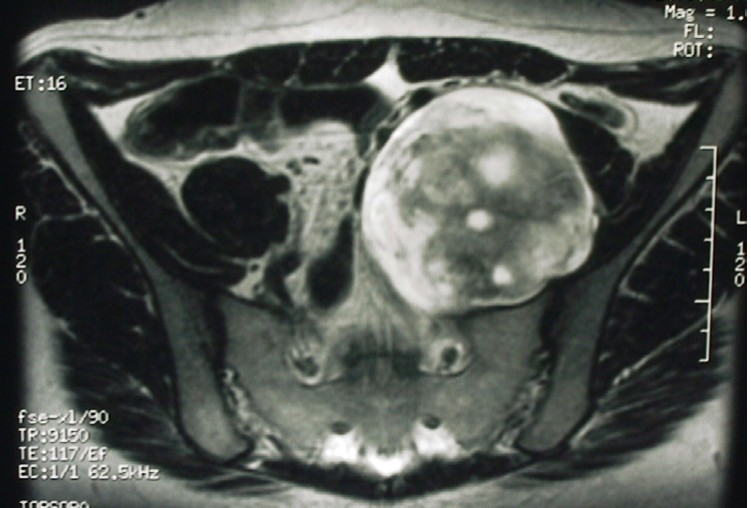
Computed tomographic scan of the pelvis showing a large, well-circumscribed presacral mass
